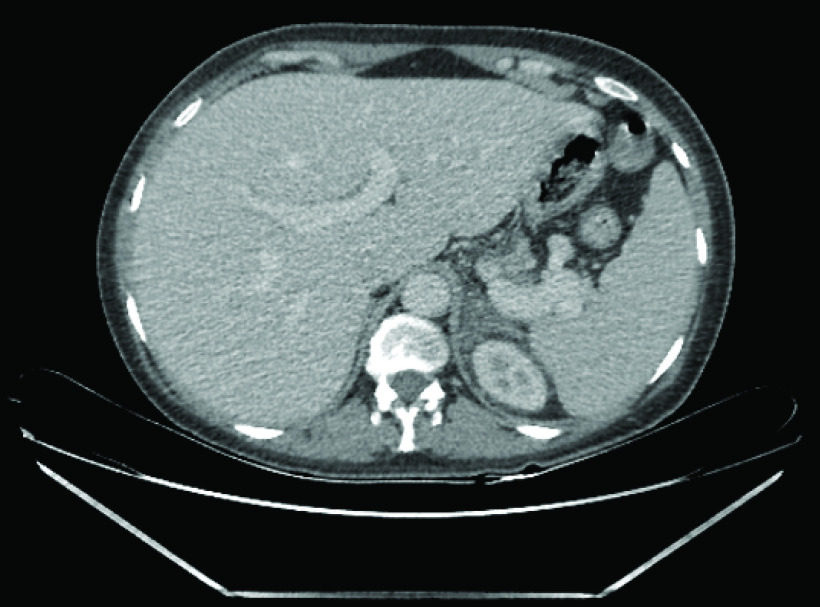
Computed tomography scan showing the left kidney and the left infarcted edematous adrenal gland in the immediate postpartum period.
